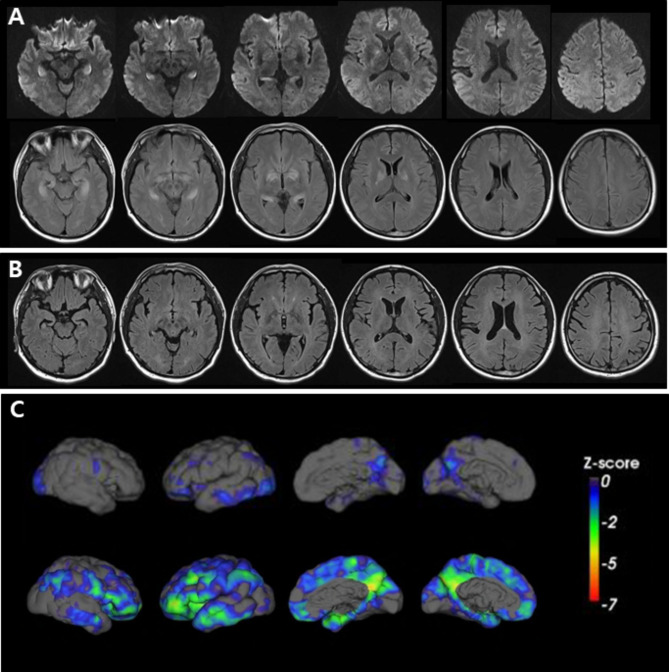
Serial brain magnetic resonance images (MRIs) and [^18^ F]-fluoro-2-deoxy-D-glucose positron emission tomographies (FDG-PETs). (A) Initial brain MRIs obtained 10 days after disappearance. Diffusion-weighted images (DWIs) show symmetric high signal intensities in the bilateral hippocampus and globus pallidus (upper row). Fluid-attenuated inversion recovery (FLAIR) images reveal prominent high signal intensities in the same lesions shown on DWIs (lower row). (B) Eight-month follow-up FLAIR images show diffuse cortical atrophy with confluent white matter hyperintensities. (C) FDG-PET mappings were obtained from the Australian e-Health Research Centre (https://aehrc.csiro.au/). The images were quantified and correlated with 3-D surface image using CapAIBL [20, 21] (https://milxcloud.csiro.au/). To quantify the uptake in PET images, focal uptake values are divided by those of the cerebellum (reference region). After quantification, a Z-score map is created on a 3-D surface image. Predominant precuneus hypometabolism in the initial map (upper row) progresses extensively to the bilateral frontoparietotemporal areas in the 8-month follow-up images (lower row)
